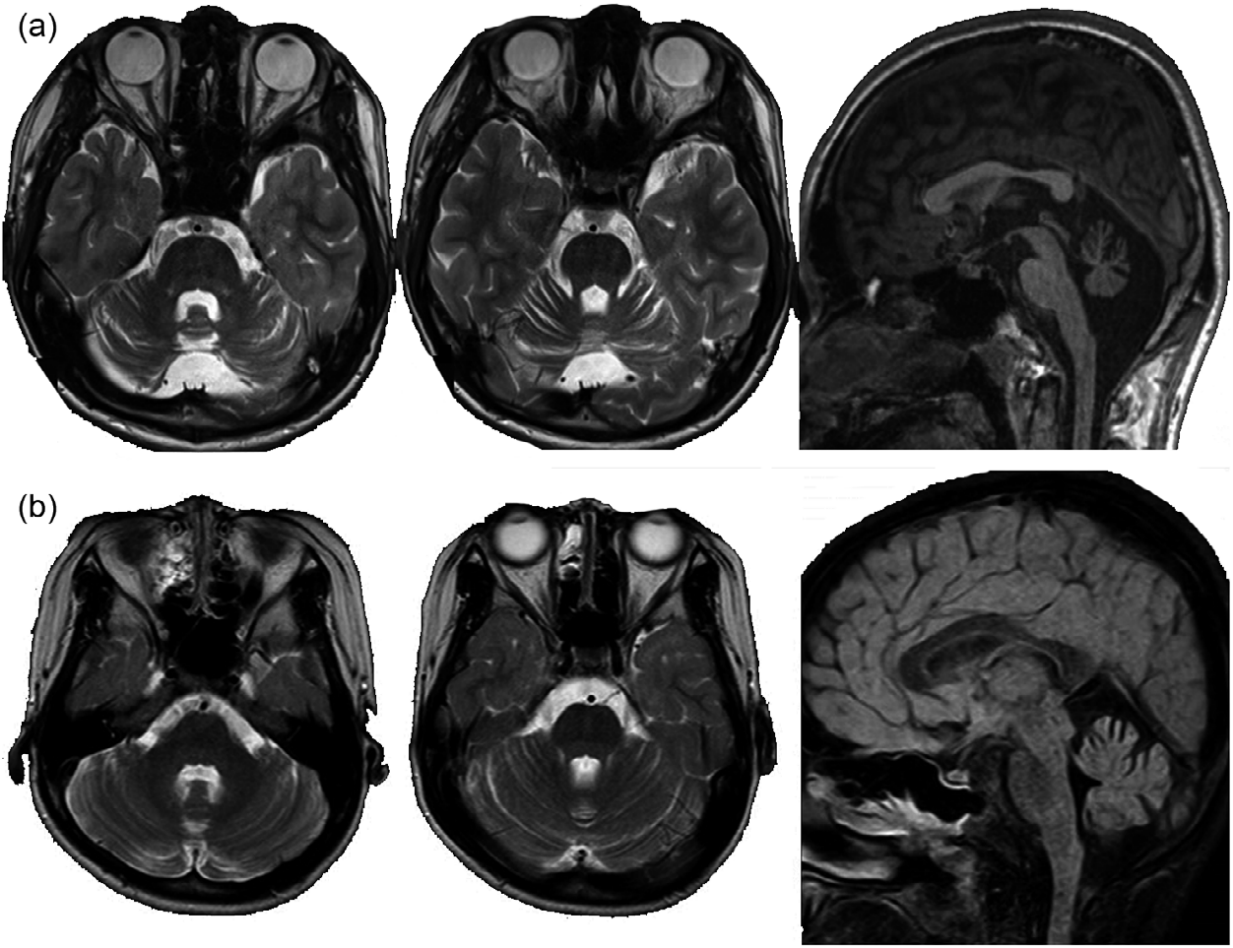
Brain magnetic resonance imaging (MRI) in case 1 (a) and Case 2 (b). (a) Brain MRI findings (axial; T2‐weighted images, coronal; short‐T1 inversion recovery, sagittal: T1) of case 1 (age 15y). Diffuse cerebellar cortical atrophy is seen predominantly in the vermis. No abnormal signal intensity is seen. (b) Brain MRI findings (axial; T2‐weighted images, sagittal; fluid‐attenuated inversion recovery) of case 2 (age 7y). Diffuse mild cerebellar cortical atrophy is seen. No abnormal signal intensity is seen.
